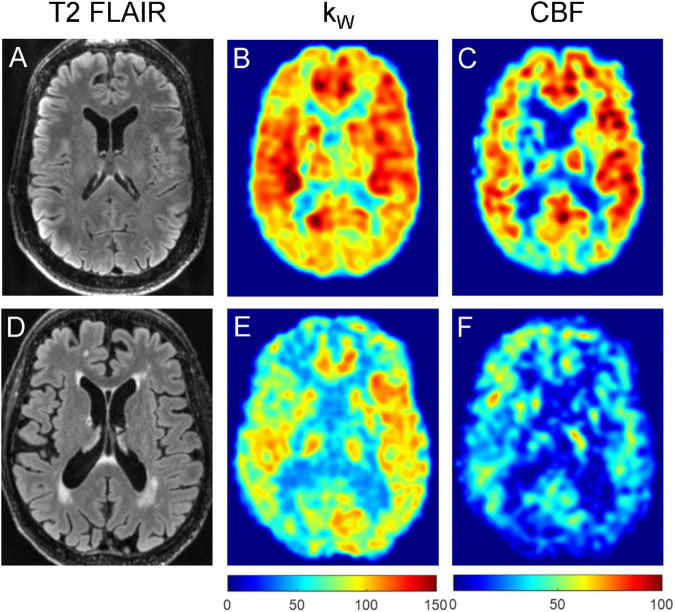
Representative T2-FLAIR images (A,D), k_W_ maps (B,E) and CBF maps (C,F) obtained from a 76-year-old subject with no vascular risk factors and no significant WMH (A–C), and a 74-year-old subject with two vascular risk factors (HTN, HLD) and moderate burden of WMH (D–F). The subject without appreciable WMH demonstrated mean cortical k_W_ of 98.4 min^–1^, mean white matter k_W_ of 93.8 min^–1^, mean cortical CBF of 56.6 mL/100 g/min, and mean white matter CBF of 47.6 mL/100 g/min. The subject with moderate WMH burden (total WMH volume of 5,456 mm^3^) demonstrated lower mean cortical k_W_ of 72.6 min^–1^, mean white matter k_W_ of 64.0 min^–1^, mean cortical CBF of 28.1 mL/100 g/min, and mean white matter CBF of 23.6 mL/100 g/min.
